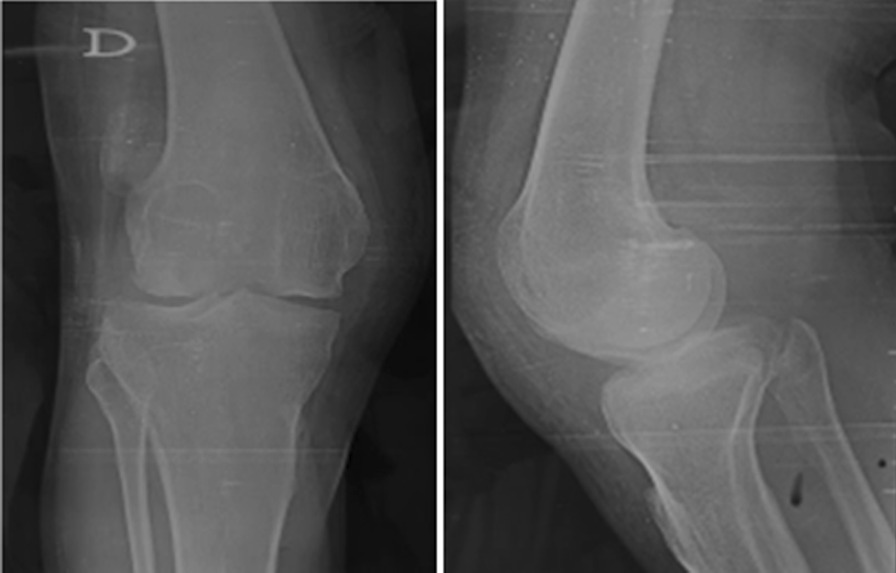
AP and lateral radiographs of the child’s father showing the laterally dislocated hypoplastic patella
